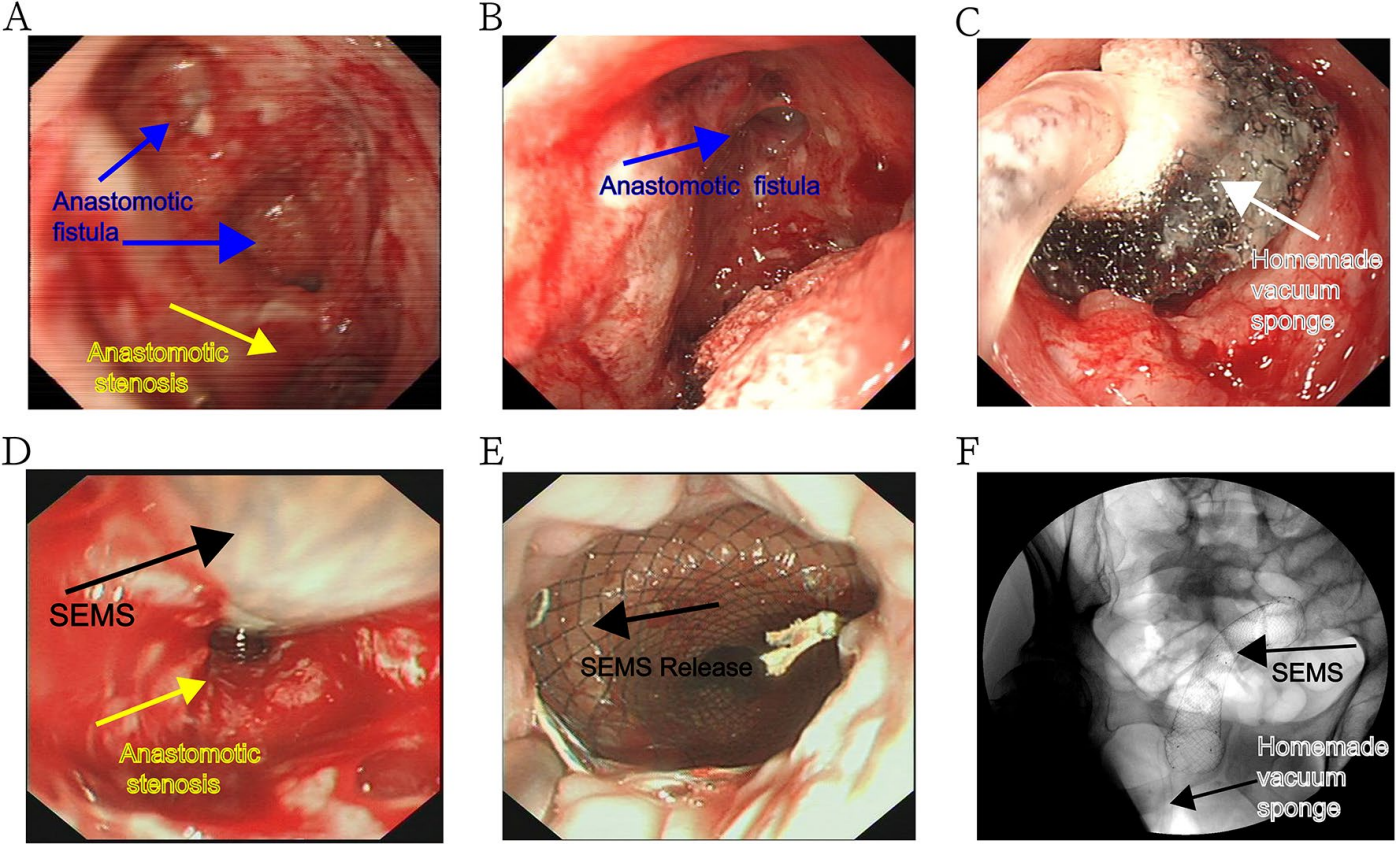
Endoscopic surgical techniques. A Anastomotic stenosis with fistula after CRC surgery. B Anastomotic fistula and its surrounding tissue. C A homemade vacuum sponge was placed at the anastomotic fistula. D Anastomotic stenosis was treated with full covered self-expanding metal stent (SEMS). E Release the SEMS. F X-ray monitor to check whether the homemade vacuum sponge and SEMS were in the correct position
